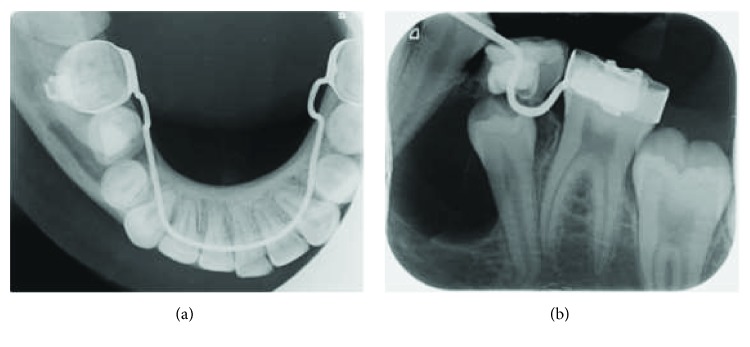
(a) Occlusal radiograph to further investigate the well-defined radiolucent lesion. (b) Periapical radiograph to further investigate the well-defined radiolucent lesion.
